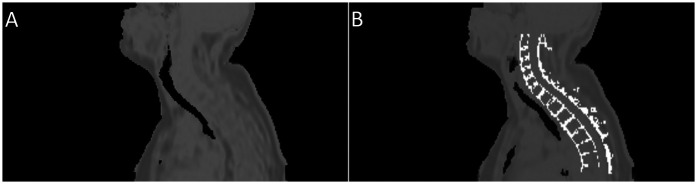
Example of the pseudo-CTs used to derive attenuation maps. Image (A) shows the pseudo-CT created from a Dixon MRI sequence by the vendor software, while image (B) shows the same psuedo-CT with segmented cortical bone from the spine added.
